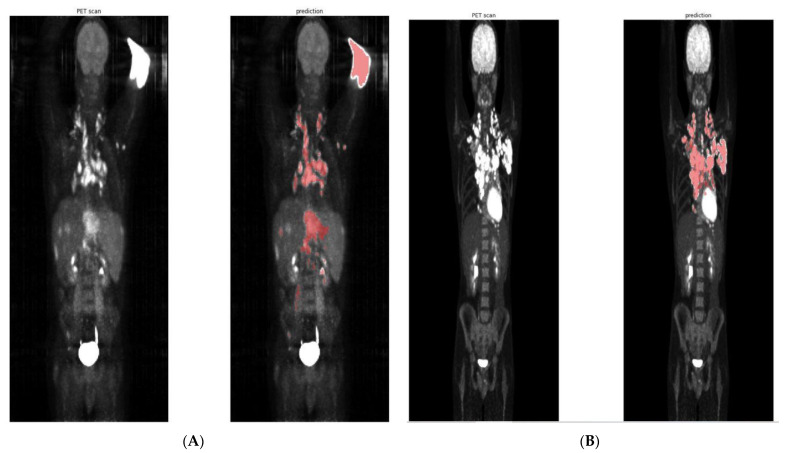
Predictions with false positives at the left arm FDG injection site, dice =0.32 (A) vs. accurate predictions, dice = 0.84 (B) of two different patients from the AHL cohort (HL).
